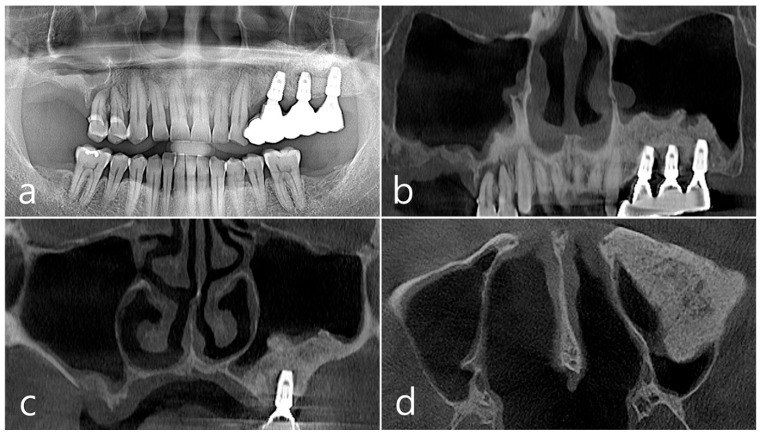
(a,b) In panoramic radiography and CBCT images taken 2 years after GBR, the sinus graft site of the maxillary sinus was well filled with bone tissue, and no abnormal radiographic images were observed; (c,d) On coronal and axial images of CBCT, homogeneous findings were observed in the previous bone defect site. Corticalization of the facial bone plate was well achieved.
